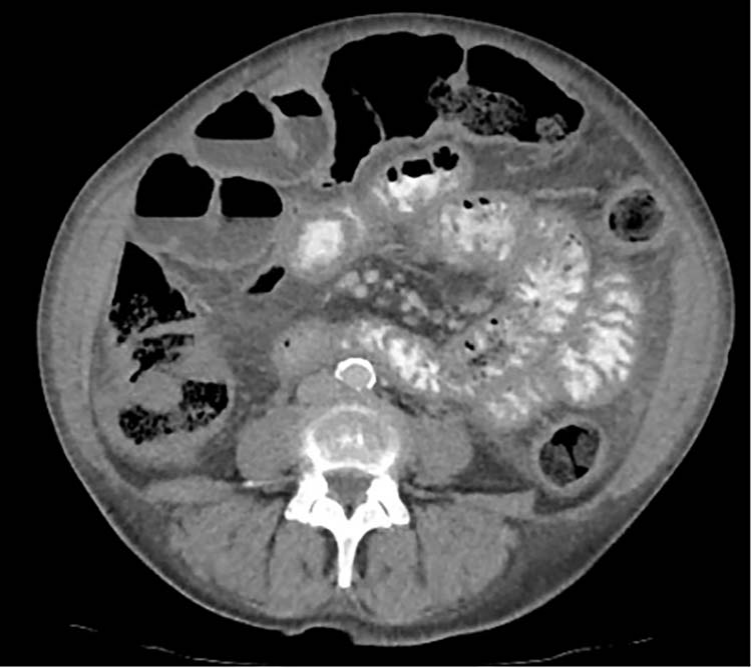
Axial CT image displaying diffuse thickening of the small and large bowel with dilatation of the small bowel.
